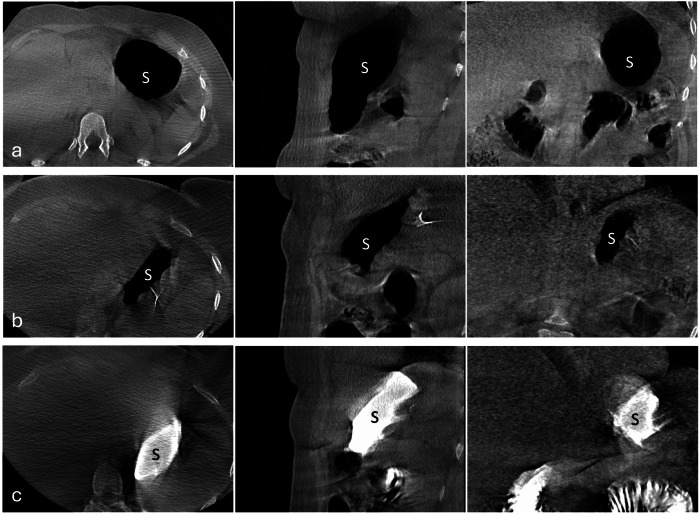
Volumetric CBCT (axial, sagittal and coronal planes, shown from left to right) acquired in the patient’s upper abdomen with CO_2_ gastric insufflation before (a) and after (b) the ESG procedure; volumetric CBCT (axial, sagittal and coronal planes, and shown from left to right) acquired with administration of a diluted Gastrografin solution after the ESG procedure (c). CBCT, Cone-beam computed tomography; S, Stomach
